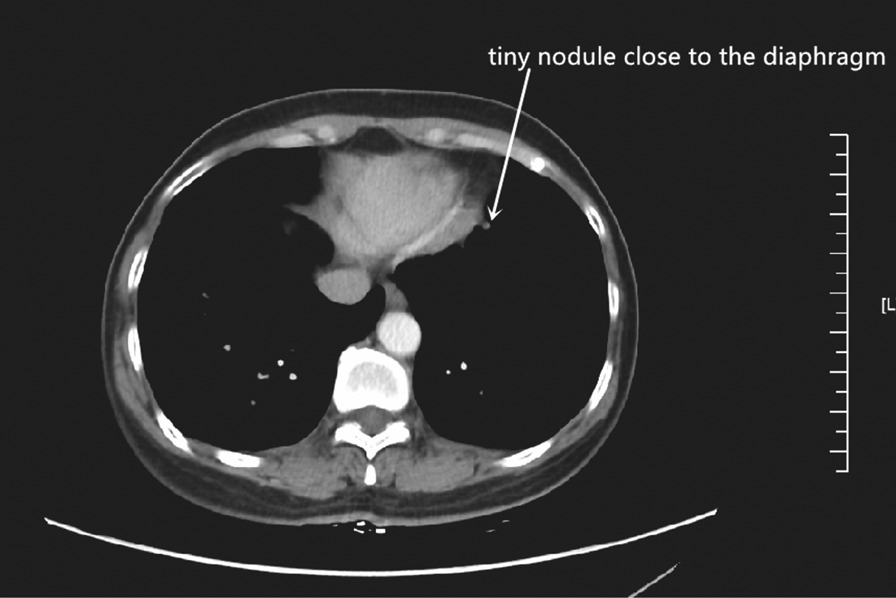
The mediastinal window on chest CT showed a tiny nodule close to the diaphragm
